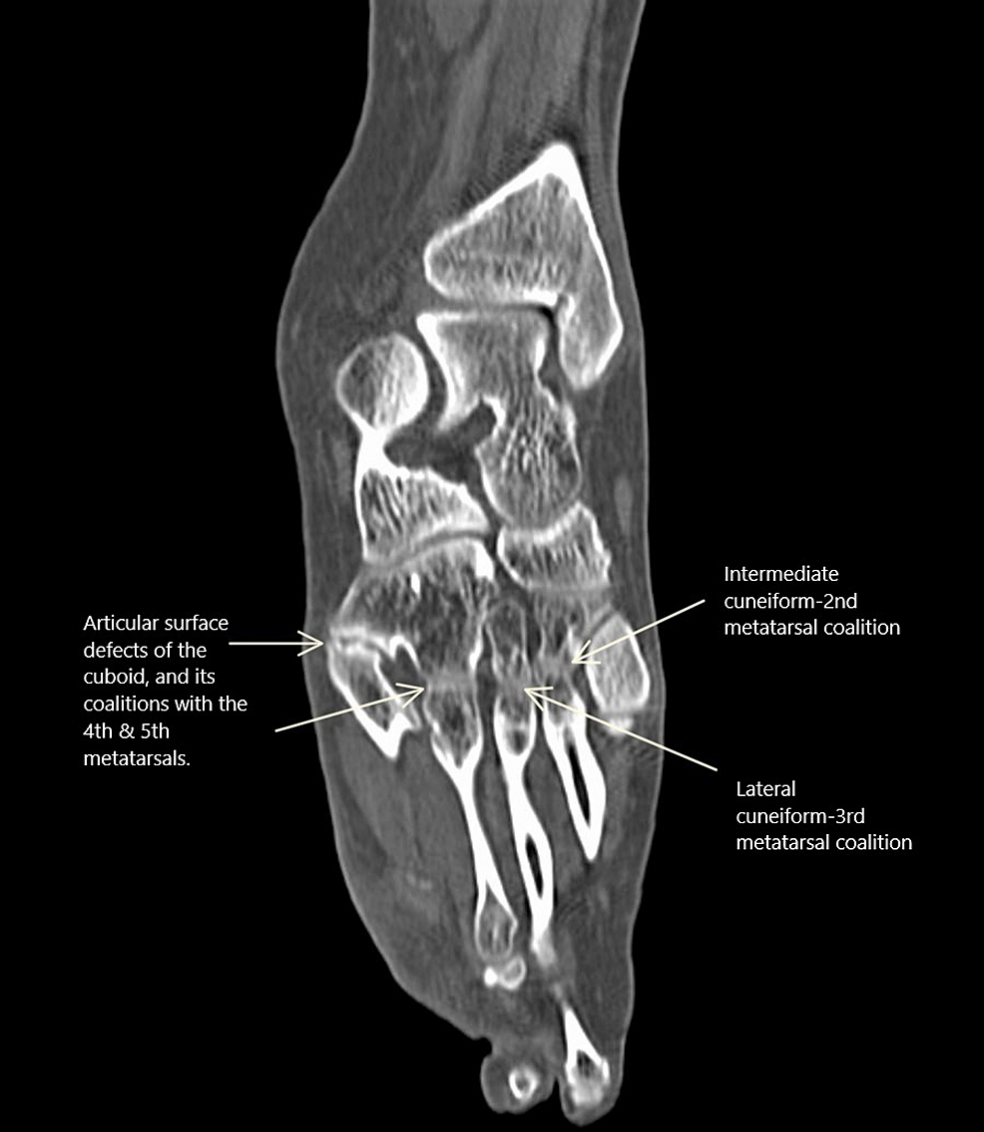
A CT scan showing the bases of the lateral four metatarsals and their respective coalitions with the cuboid, lateral, and intermediate cuneiforms.
